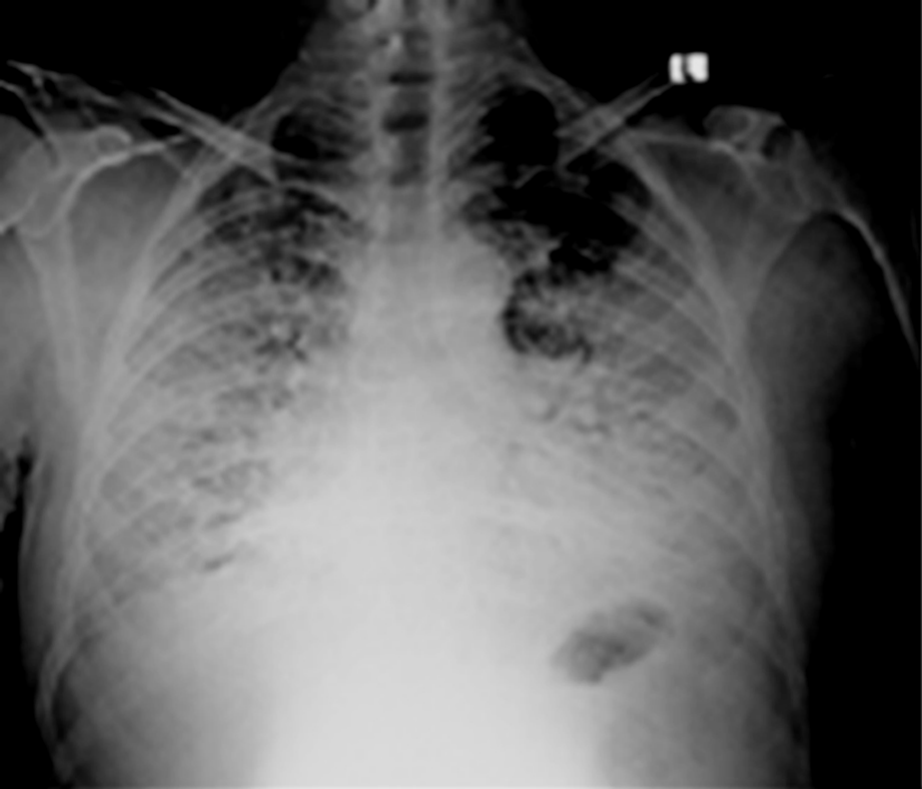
Chest X-ray of a COVID positive, middle aged female patient showing homogenous consolidation in bilateral lung fields predominantly in peripheral distribution in mid and lower zones with obscuration of cardiac and diaphragmatic silhouette and costophrenic angles on both sides. Patient had an acute episode of fever, shortness of breath and cough with no positive contact history.
